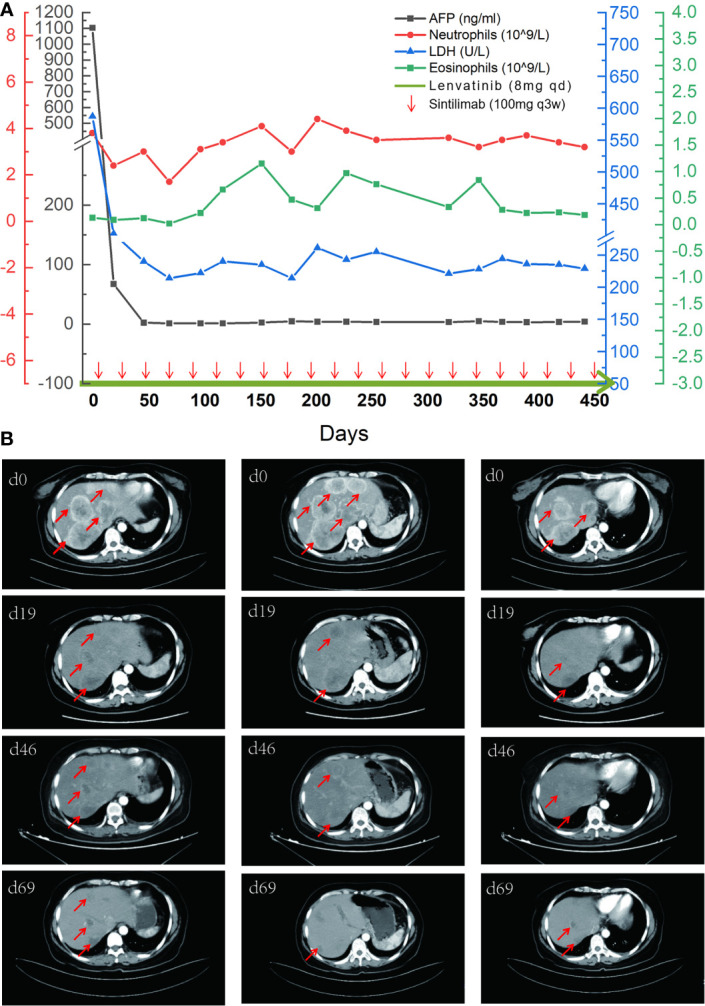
The comparison of tumor indicators and CT images. (A) A timeline outlining the treatment course (lenvatinib 8 mg, orally, qd; and sintilimab 100 mg, intravenous glucose tolerance test, q21d) and changes of laboratory indicators including alpha-fetoprotein (AFP), neutrophils, lactate dehydrogenase (LDH), and eosinophils. (B) Imaging changes of the first four times of evaluation with tumor lesions marked by red arrows.
